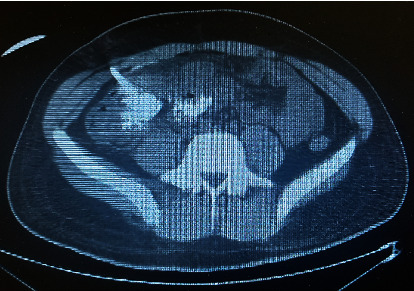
CT-guided drainage of the 10 cm abscess collection near the appendix.
